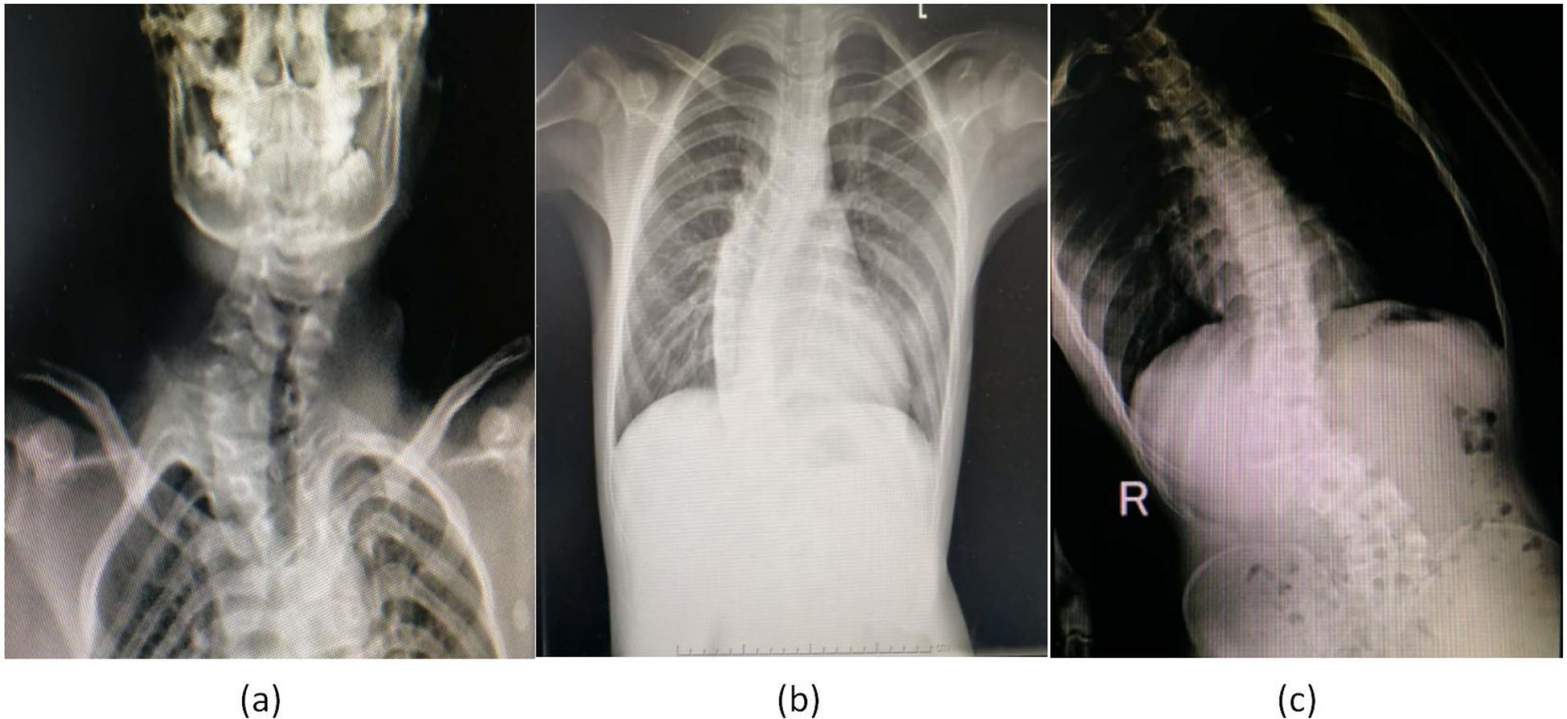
The CT images of the pediatric patients with congenital scoliosis. Cervical lateral bending (a); thoracic scoliosis (b); lumbar scoliosis (c)
